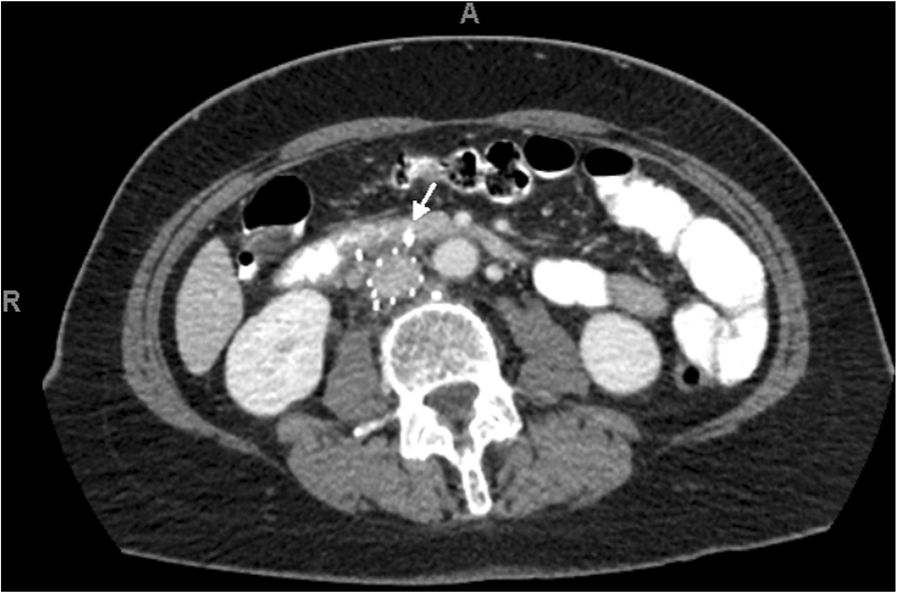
Computed tomography scan Abdomen (axial) showing struts of filter extending to adjacent structures after penetrating the wall of inferior vena cava. Arrow pointing to one of the anterior struts of inferior vena cava filter penetrating the duodenum
